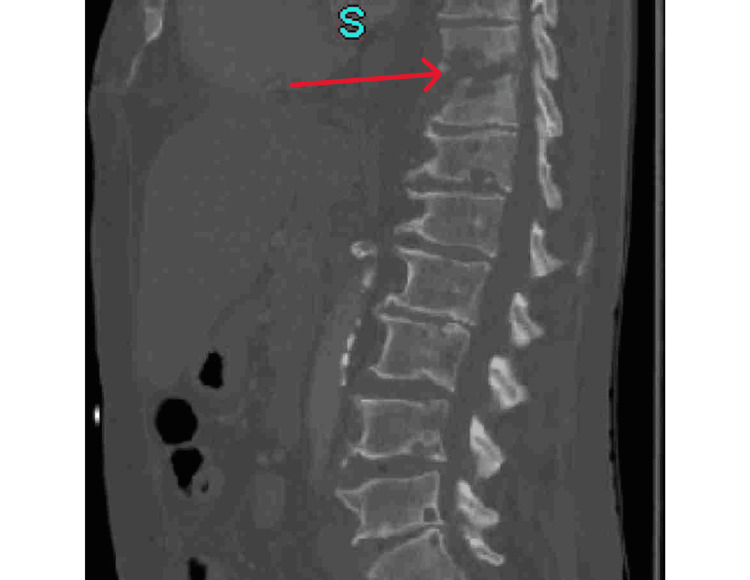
Contrast-enhanced CT scan of the abdomen and pelvis Extensive lytic changes involving the T10 and T11 vertebra, centered around the disc space, with perivertebral soft tissue density. Findings concerning for osteomyelitis/discitis.
